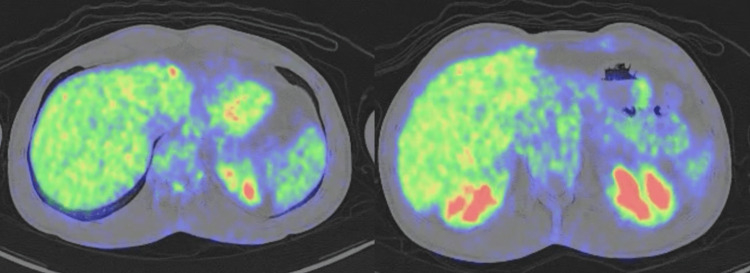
Follow-up FDG PET/CT after hepatic resection and immunotherapy Post-treatment fluorodeoxyglucose (FDG) PET/CT scan performed during follow-up after hepatic resection and nivolumab therapy, demonstrating no abnormal hypermetabolic activity suggestive of residual or recurrent metastatic disease. PET/CT: Positron emission tomography/computed tomography.
